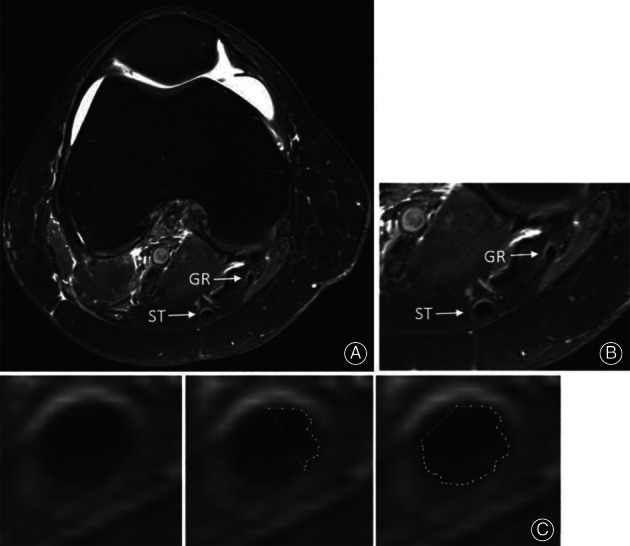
Magnetic resonance image (MRI) image for hamstring tendon cross‐sectional area (CSA) measurement. (A) Axial view of the right knee at the widest point of the medial femoral condyle. (B) Magnified view at the same level showing the semitendinosus (ST) and gracilis (GR) tendons. (C) Demonstration of the use of the region‐of‐interest tool to trace the cross‐sectional area of the ST tendon^41^.
